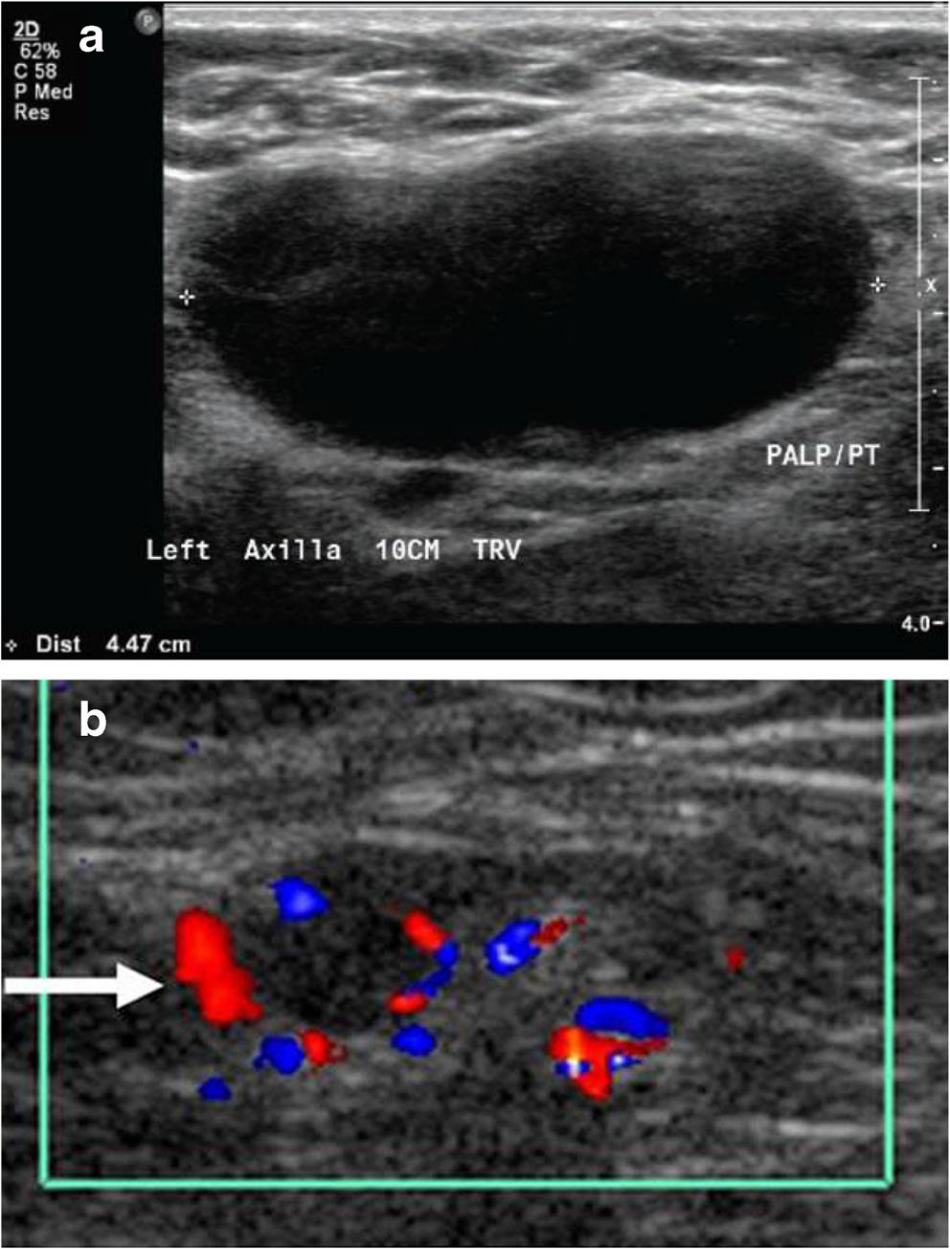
Abnormal lymph nodes: characteristics concerning for malignancy: a absence of the fatty hilum and b increased focal cortical thickness greater than 3 mm with colour Doppler US that shows hyperaemic blood flow in the hilum and central cortex or abnormal (non-hilar cortical) blood flow
